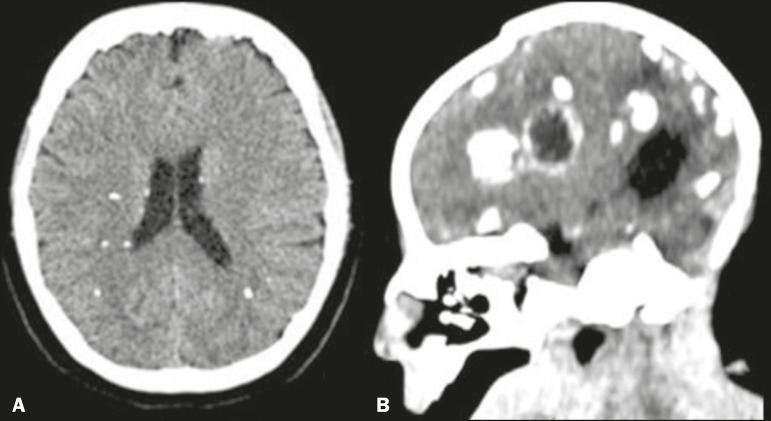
Unenhanced axial and sagittal cranial CT scans (A and B, respectively), with soft-tissue window settings, showing periventricular and particularly subcortical calcifications, in a case of neurocysticercosis (A), as well as periventricular and cortical (sometimes rope-like), calcifications in a child with microcephaly with an apparently “collapsed” skull, in a case of congenital Zika virus infection (B).
